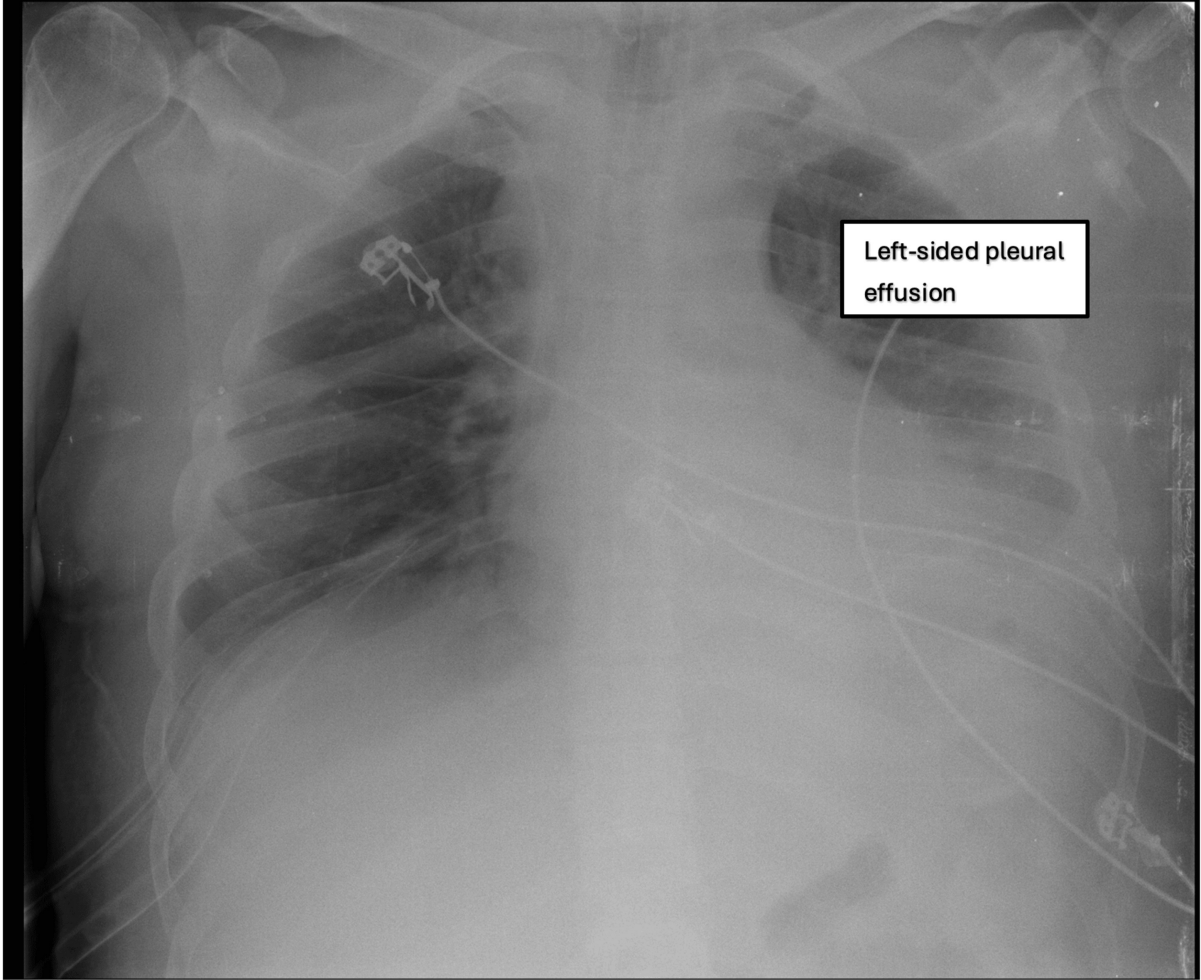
Chest X-ray showing new, left-sided pleural effusion on the day of transfer to the MICU (day 6 of hospitalization). MICU: medical intensive care unit
